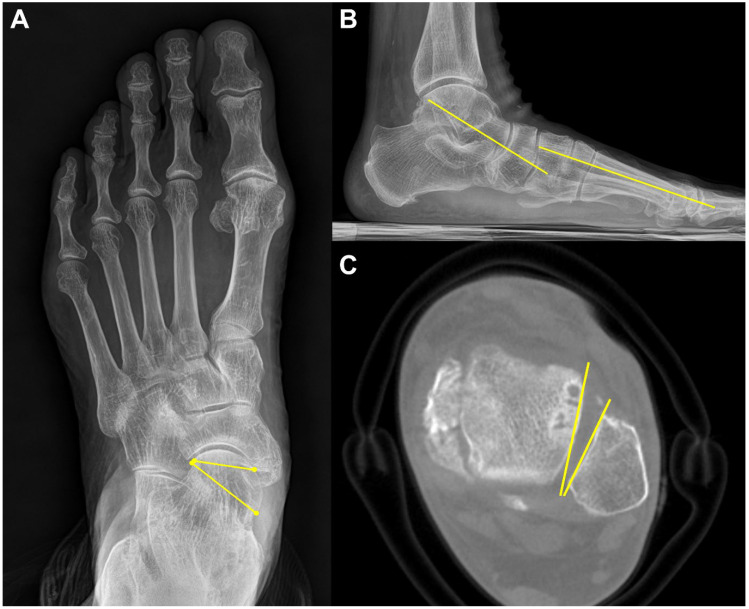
A 71-year-old male PCFD patient with 35-degree talonavicular coverage angle (A) and 20-degree lateral Meary’s angle (B). The talus is internally rotated with regard to lateral malleolus on the weight-bearing CT axial image (C).
